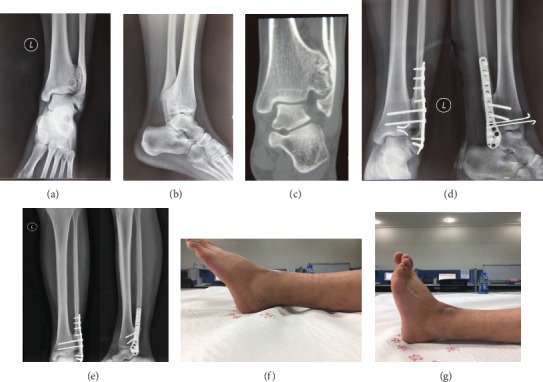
Preoperative ((a) anteroposterior; (b) lateral; (c) CT scan with one layer) and postoperative ((d) day 1 after surgery; (e) 6 weeks after surgery, the Kirschner wires have been removed) radiographs from a 32-year-old man. It is noted that the posterior tibial osteotomy was used to retract the Volkmann tuberosity, which was reduced back anatomically to preserve the inferior tibiofibular syndesmosis intact. The lateral photograph at the final follow-up showed good range of motion of ankle joint (FnnG).
